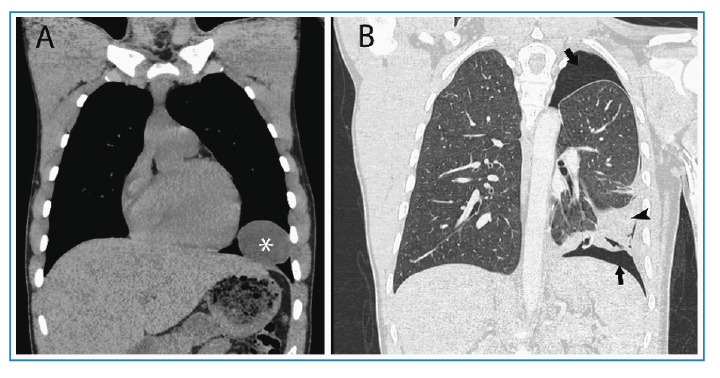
Non-contrast coronal thoracic computed tomography (A) showing a well-defined cystic lesion in the lower lobe of the left lung in the mediastinal window (asterisk). The cyst density measured to be 10 HU. Contrast-enhanced coronal thoracic computed tomography (B) demonstrating air densities consistent with pneumothorax between the pleural layers in the parenchymal window (black arrows). Additionally, marked wall irregularity and pleuropulmonary fibroatelectatic changes with volume loss are observed at the previously identified location of the cyst in the lower lobe of the left lung (black arrowhead).
